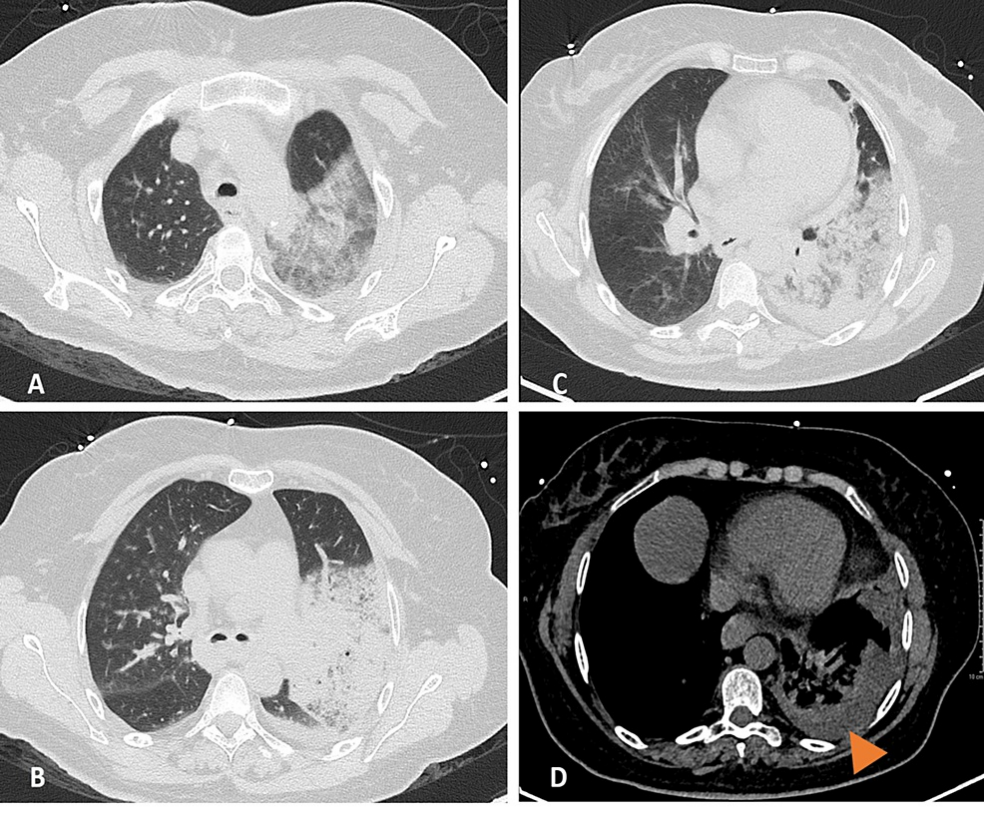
Chest computed tomography performed on the day of admission. Axial unenhanced computed tomography images in the lung window at the apex (A), mid-lung (B), and base (C) demonstrate areas of consolidation in the left upper and lower lobes, with air bronchogram and surrounding ground glass opacities. Axial unenhanced computed tomography image in a mediastinal window at the base (D) shows a small left pleural effusion (arrowhead).
